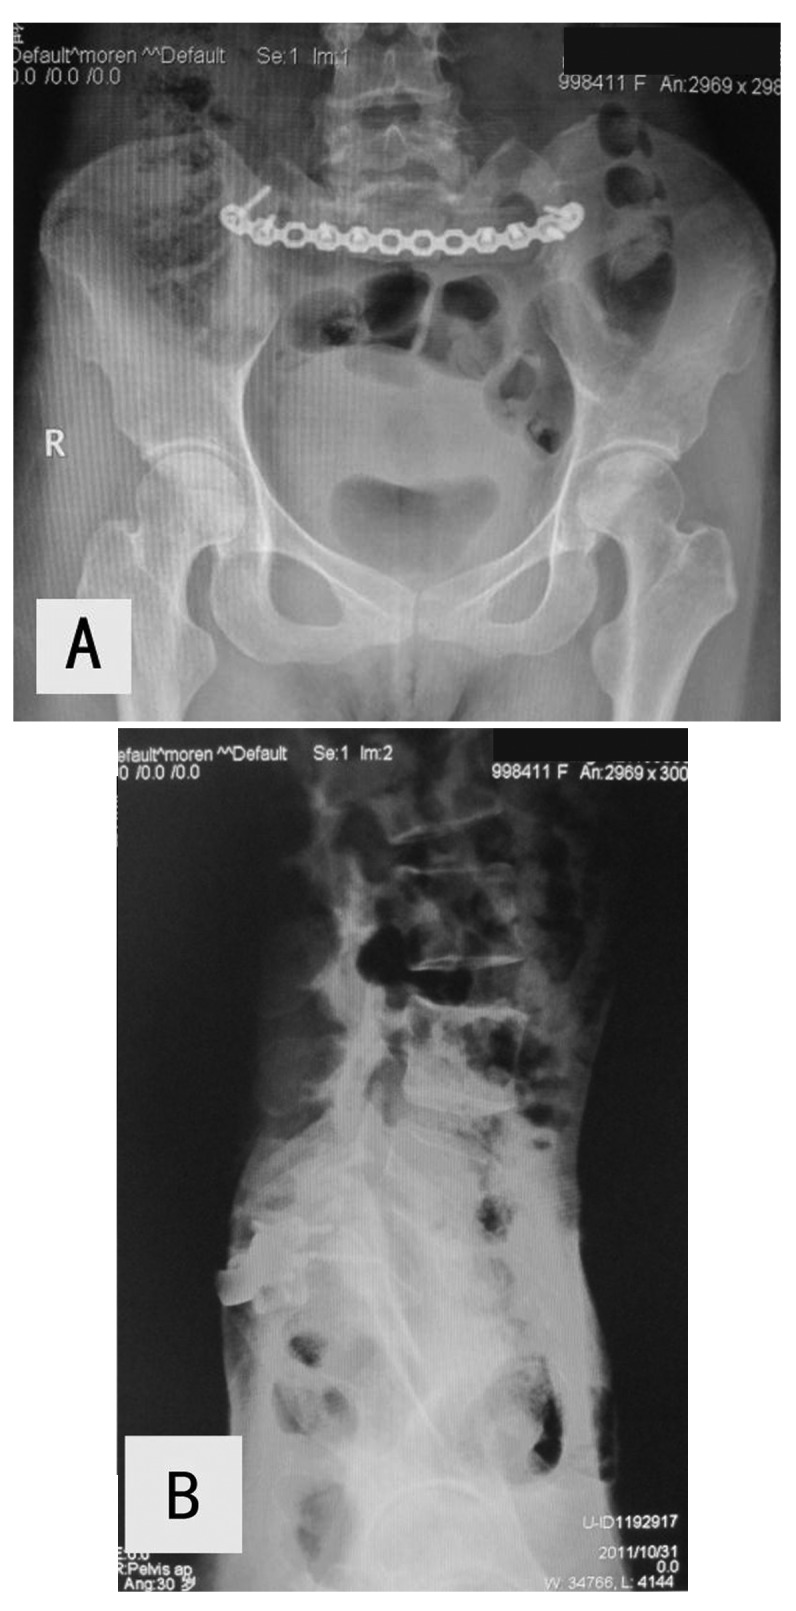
Plain X-ray of the (A) pelvic and (B) sacroiliac regions six months after surgery, neither of which exhibited any looseness or fractures of internal fixation or new bone destruction.
